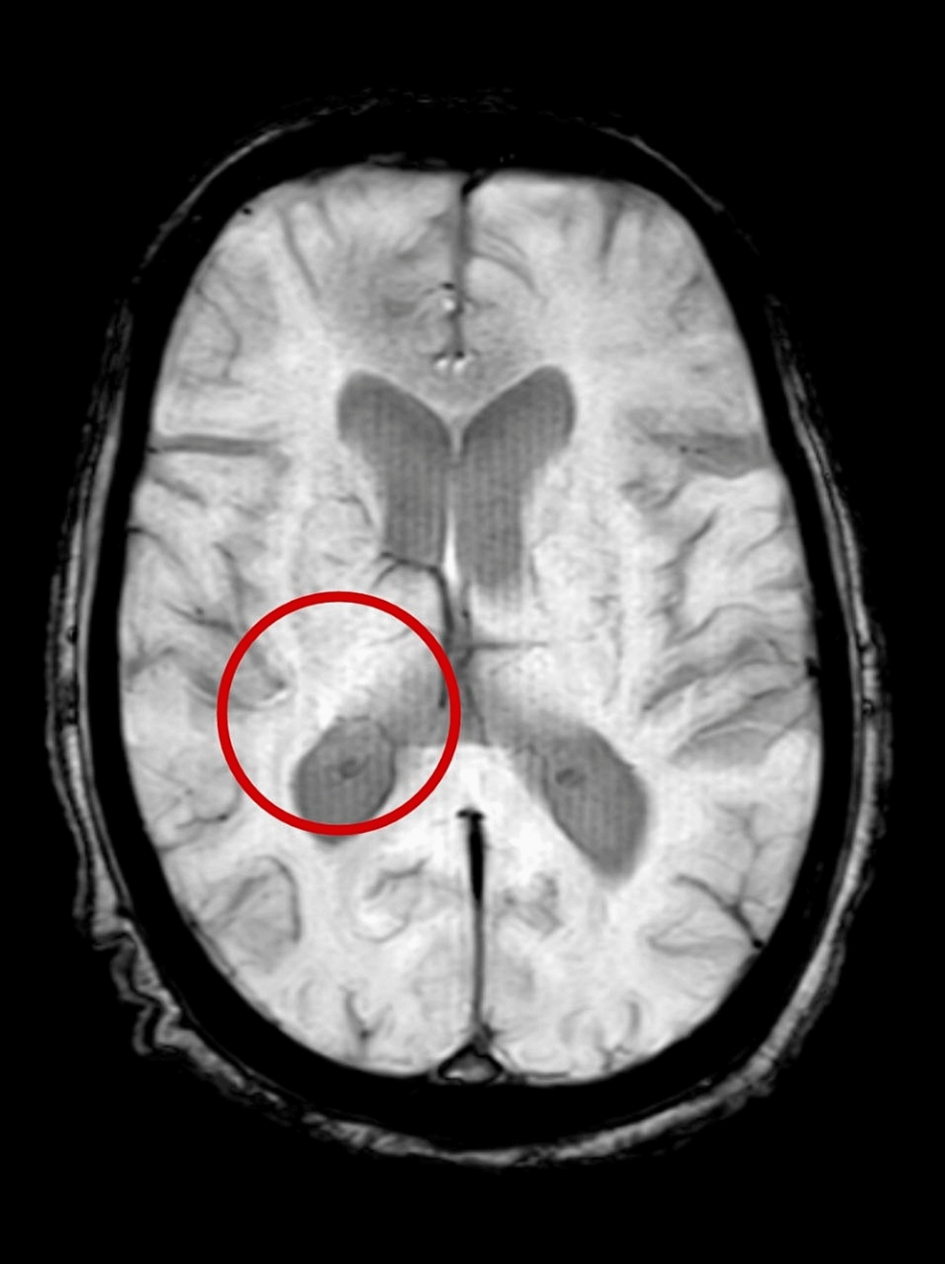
Magnetic resonance imaging findings The red circle indicates small vessel ischemic changes involving bilateral frontoparietal periventricular white matter with Fazekas grade 1, along with age-related cerebral atrophy.
